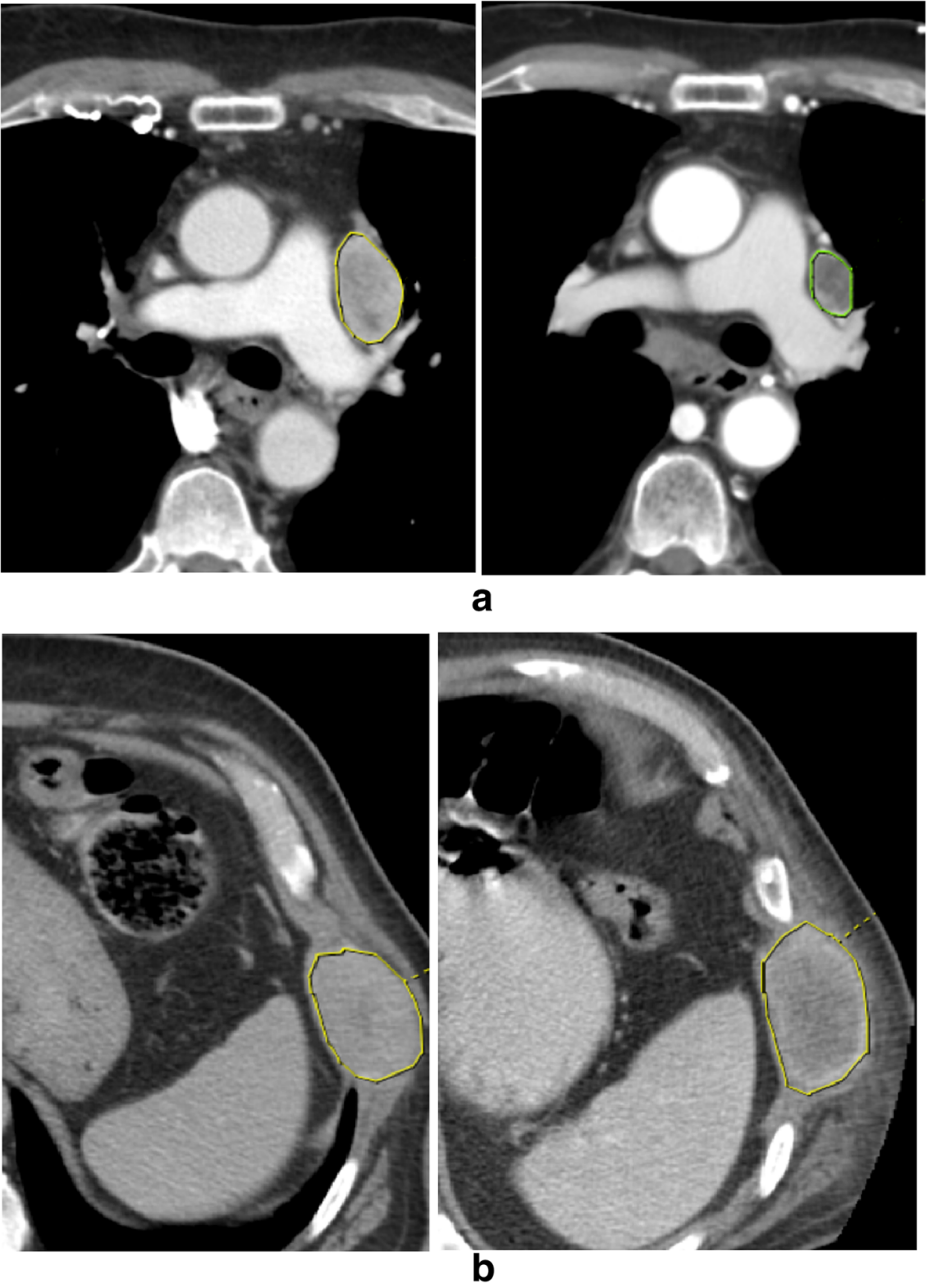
Baseline CT in patients with metastatic clear cell renal cell carcinoma and intermediate IMDC score (Left: prior to treatment, Right: following the treatment). a 72-year-old man: OS and PFS in this patient was 1074 and 1063 days, respectively with a baseline nSD = 0.66. Although the tumor remains heterogeneous in appearance there is response by RECIST criteria. b 40-year-old man: OS and PFS in this patient were poor at 159 and 113 days, respectively with a lower baseline nSD of 0.54. The tumor is slightly larger but stable by RECIST criteria. The list of significant parameters for 2 cases is shown in Table 6 below. As it can be seen from the table, higher nSD prior to and following treatment and higher percent change in lesion size (i.e., higher size reduction) were associated with higher survival; and higher entropy following treatment and entropy change predicted decreased survival. It should be noted that for PFS, only nSD prior to and following treatment were statistically significant parameters
